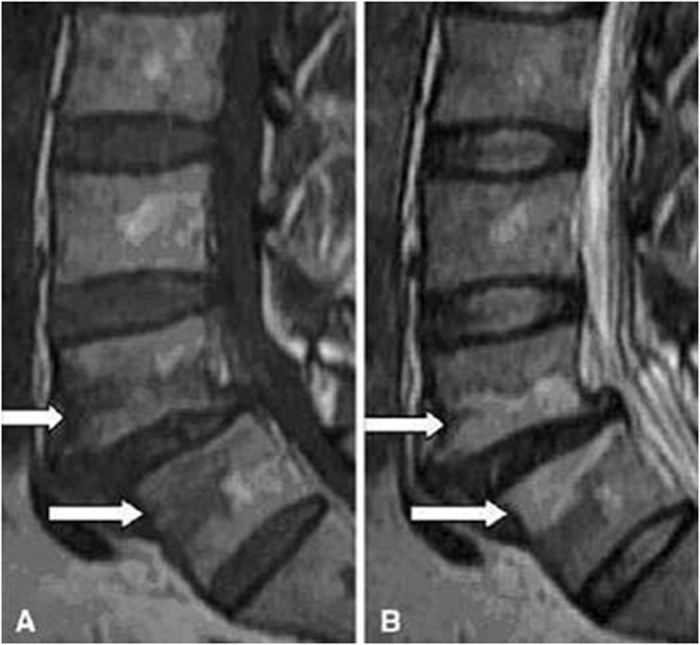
Magnetic resonance images of type I MCs at the L4–5 level in the lumbar spine.
